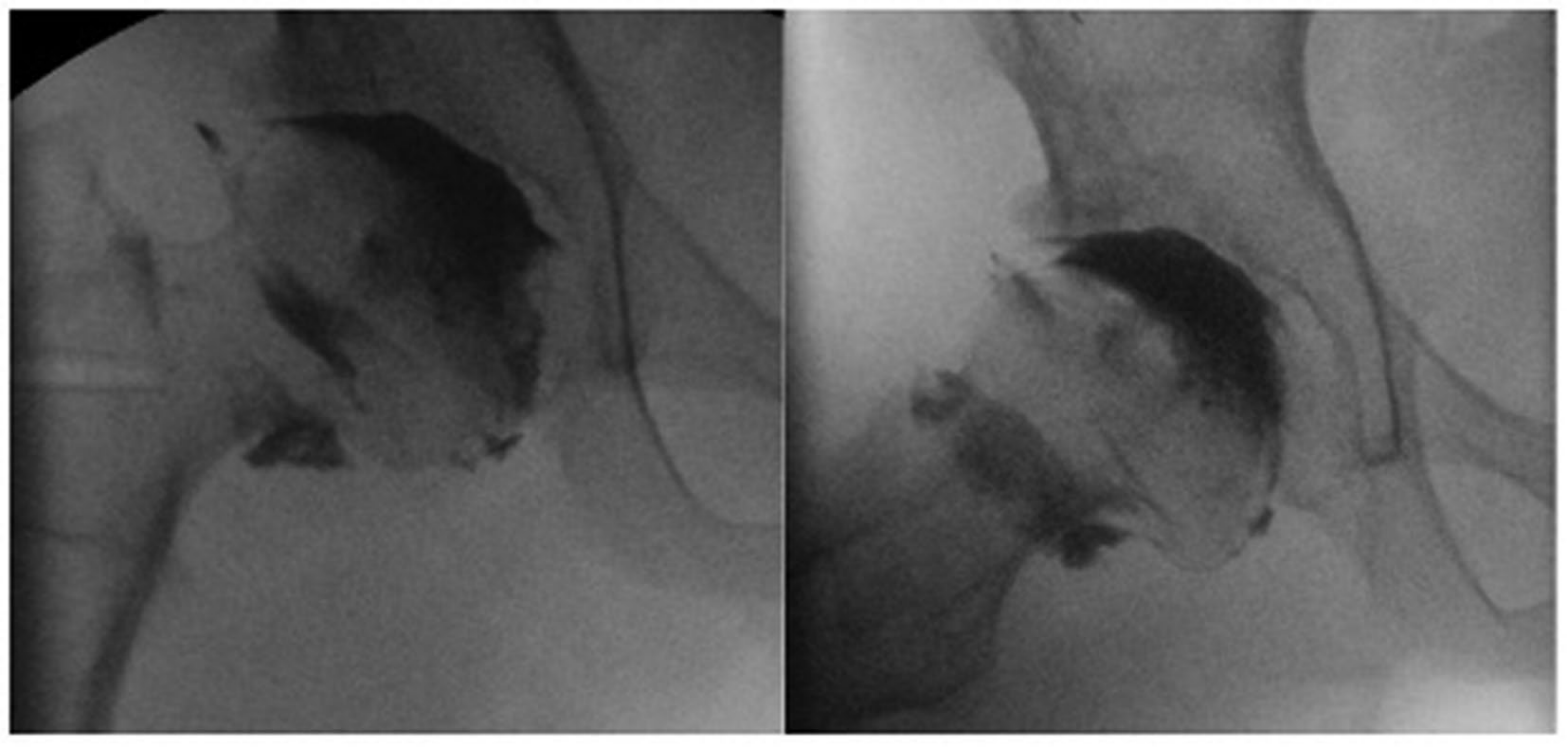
Arthrography: The affected hip joint is visualized in more detail by intra-articular application of contrast medium. These functional images provide a reliable method, in addition to x-ray in abduction, for detecting hinge abduction prior to planned surgery. Furthermore, arthrography provides reliable assessment of the containment and congruence of the head and cup
